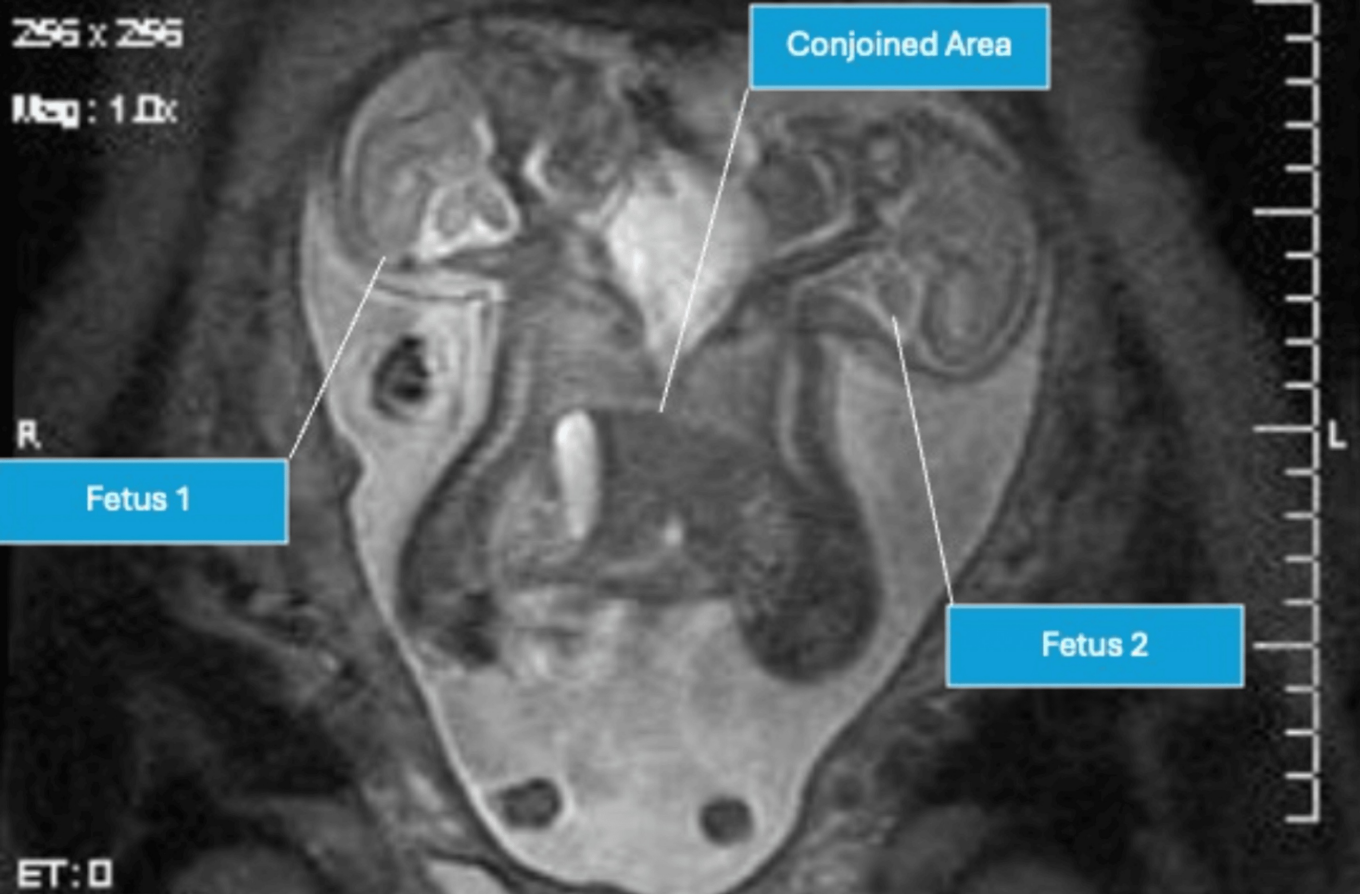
MRI image depicting the thoracic attachment of the conjoined twins, as indicated by the blue boxes.
